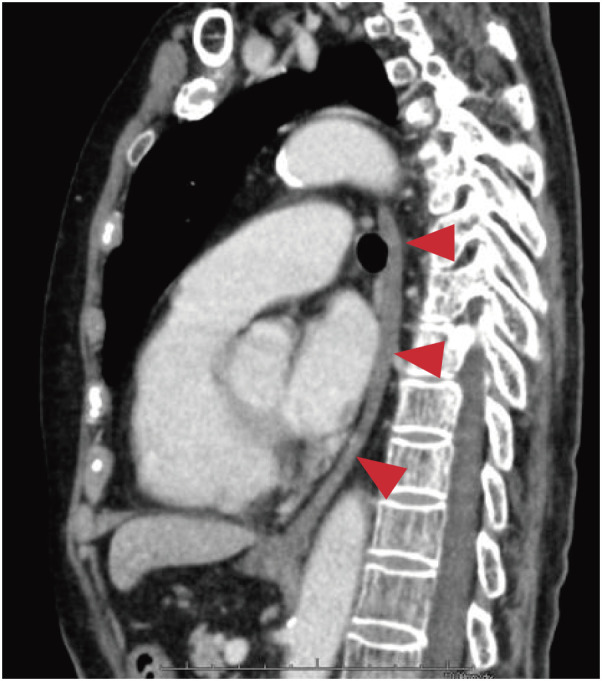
Contrast-enhanced computed tomography of the thorax and abdomen. From the cervical to the abdominal esophagus, the tissue is scarred and has a cord-like shape (arrowheads).
